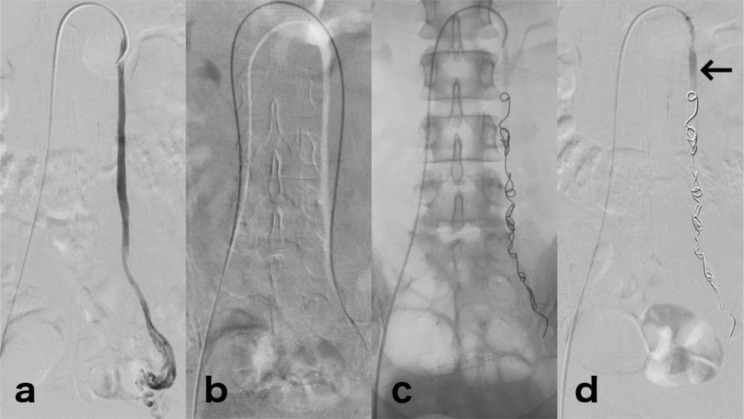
Embolization technique. (a) Venography pre-embolization with reflux in the left ovarian vein to periuterine veins. (b) Left ovarian vein catheterization to its distal segment using angiographic catheter over a hydrophilic guide wire. (c) Radiography after embolization, showing fibered metallic coils from the distal segment of the left ovarian vein to its proximal third. (d) Venography post-embolization, with complete occlusion of the vessel. Note the contrast material only in the proximal segment of the left ovarian vein (arrow).
